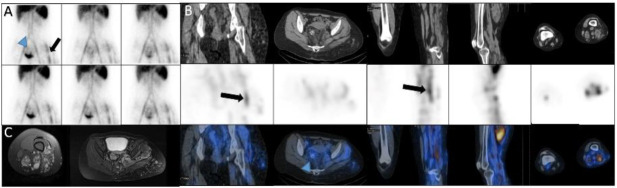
Dynamic (A) and SPECT/CT images (B) showed soft tissue hemangiomata (black arrows). MRI confirmed hemangioma (C). Source of bleeding was a rectal hemangioma (arrow head)
